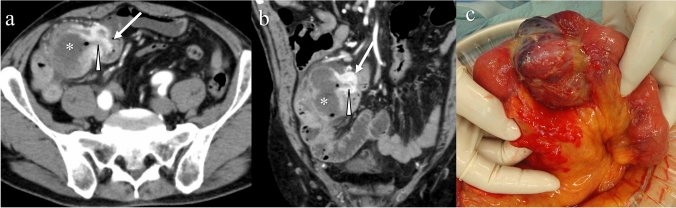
Ruptured gastrointestinal stromal tumor in the small bowel. A 72-year-old male presented with epigastralgia and his pain gradually migrated from the upper abdomen to the right lower abdomen, resembling a symptom of acute appendicitis. Axial (a) and coronal (b) images of contrast-enhanced CT in the arterial phase showing the hypervascular tumor (arrows) in the small bowel and adjacent fluid collection (asterisks) with fistula (arrowheads). Laparotomy showing that the tumor ruptured into the mesentery (c)
